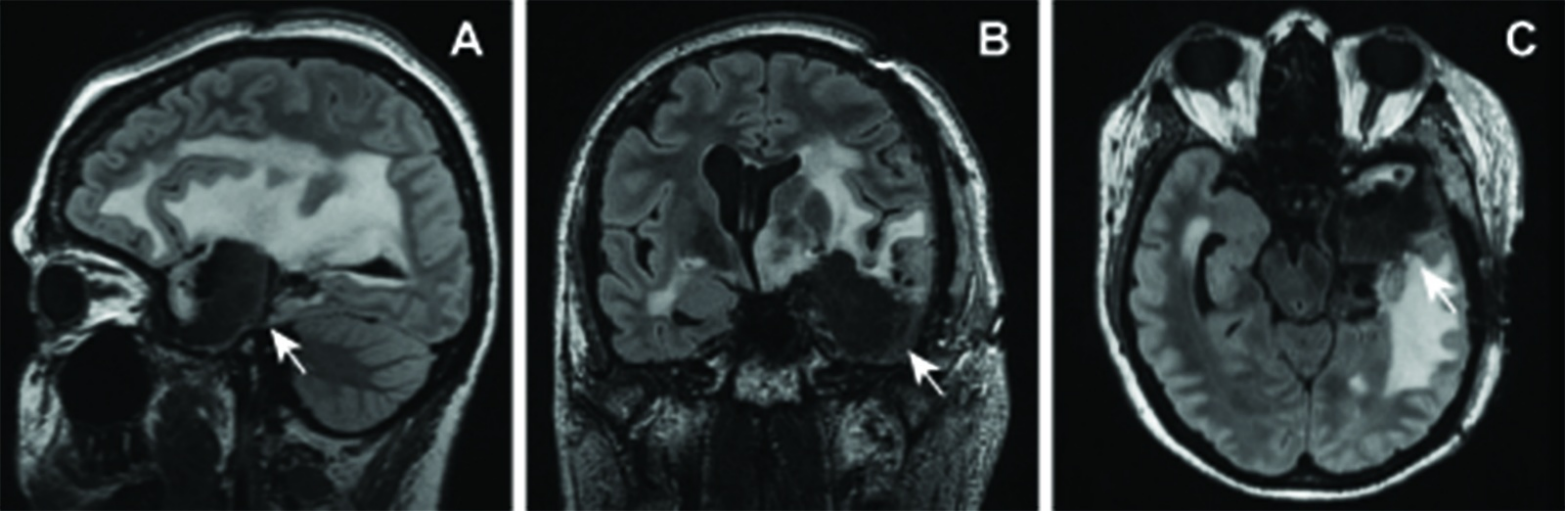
Postoperative MRI Sagittal (A), coronal (B), and axial (C) FLAIR images demonstrating interval left frontotemporal craniotomy with gross total resection of the cavernous malformation and anterior temporal lobectomy with expected postoperative changes. Arrows, region of interest.
